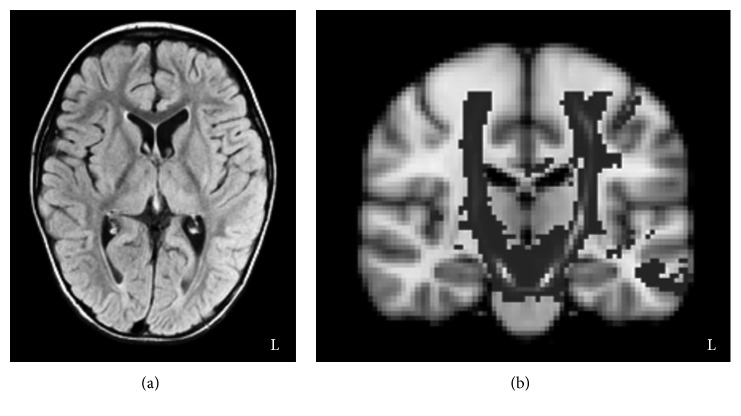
Brain magnetic resonance and fiber tracking images. (a) A brain magnetic resonance image at age 6 showing the mild degree of cerebral atrophy. (b) A fiber tracking image reconstructed corticospinal tract in both hemispheres.
